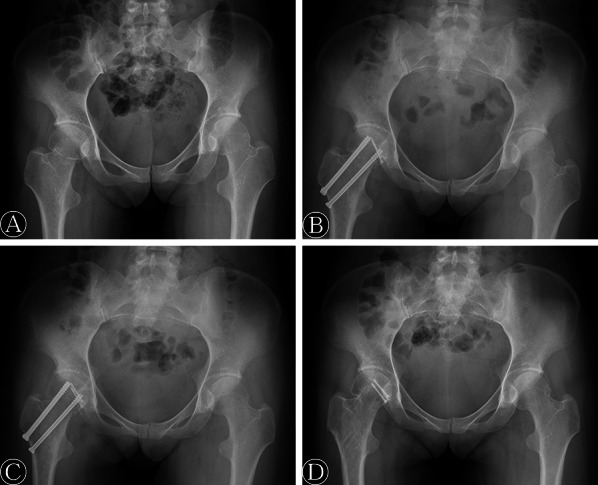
A 28-year-old woman with a right Pipkin type III femoral head fracture. A Preoperative anteroposterior radiograph of the pelvis. B Anteroposterior radiograph of the pelvis after open reduction and internal fixation. C Anteroposterior radiograph of the pelvis at 3 months postoperatively, demonstrating bony union. D Radiograph at 46 months after surgery
